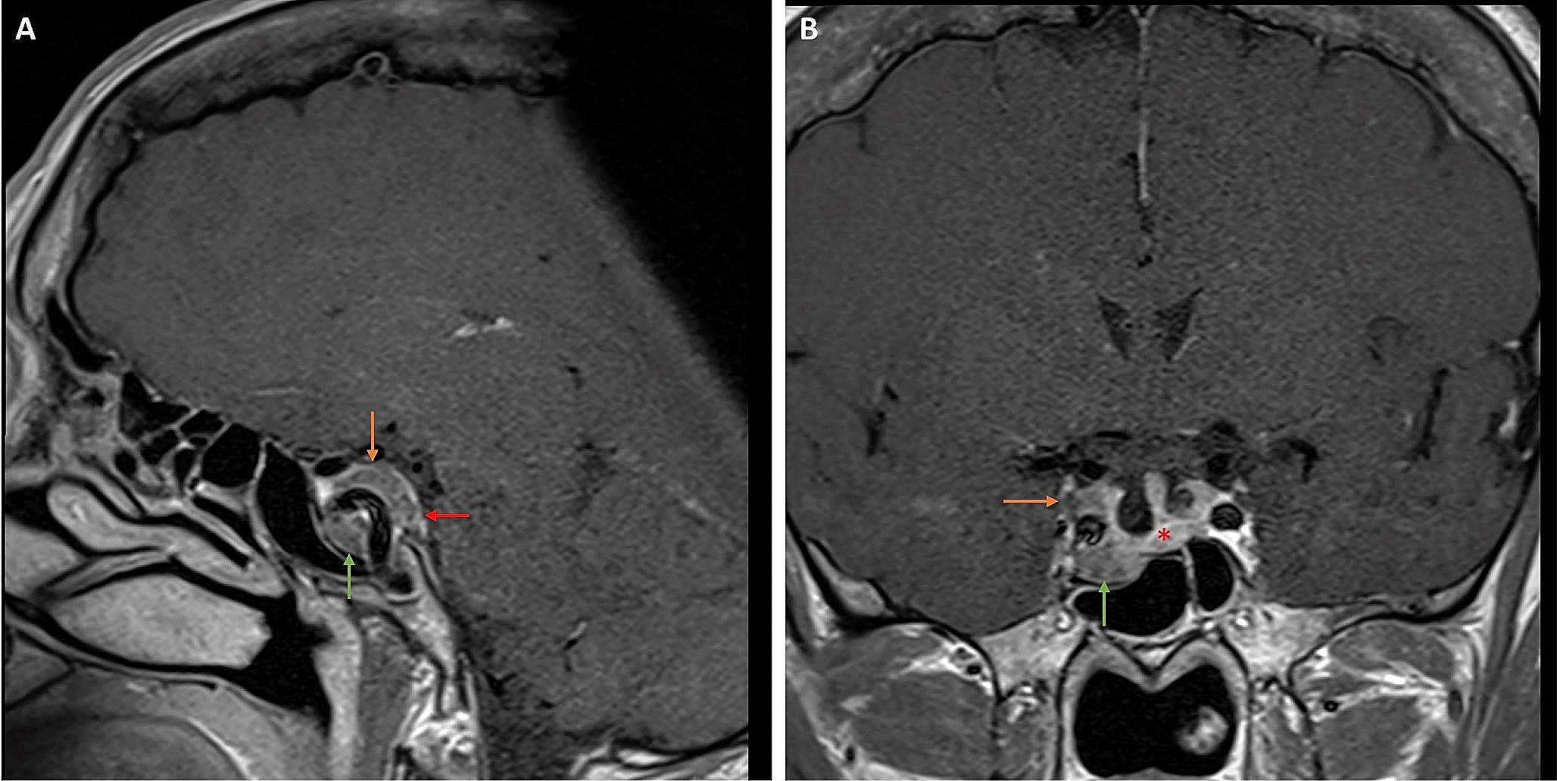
Case involving a 62-year-old patient with abnormal IGF levels (745 µg/L) and acromegalic features. (A and B) Pre-operative Gd-enhanced T1W sagittal and coronal MR images showing a pituitary macroadenoma invading the inferior (green arrow), medial, superior (orange arrow) and posterior (red arrow) compartments of the right cavernous sinus. Pituitary gland (red star) is slightly displaced to the left side
